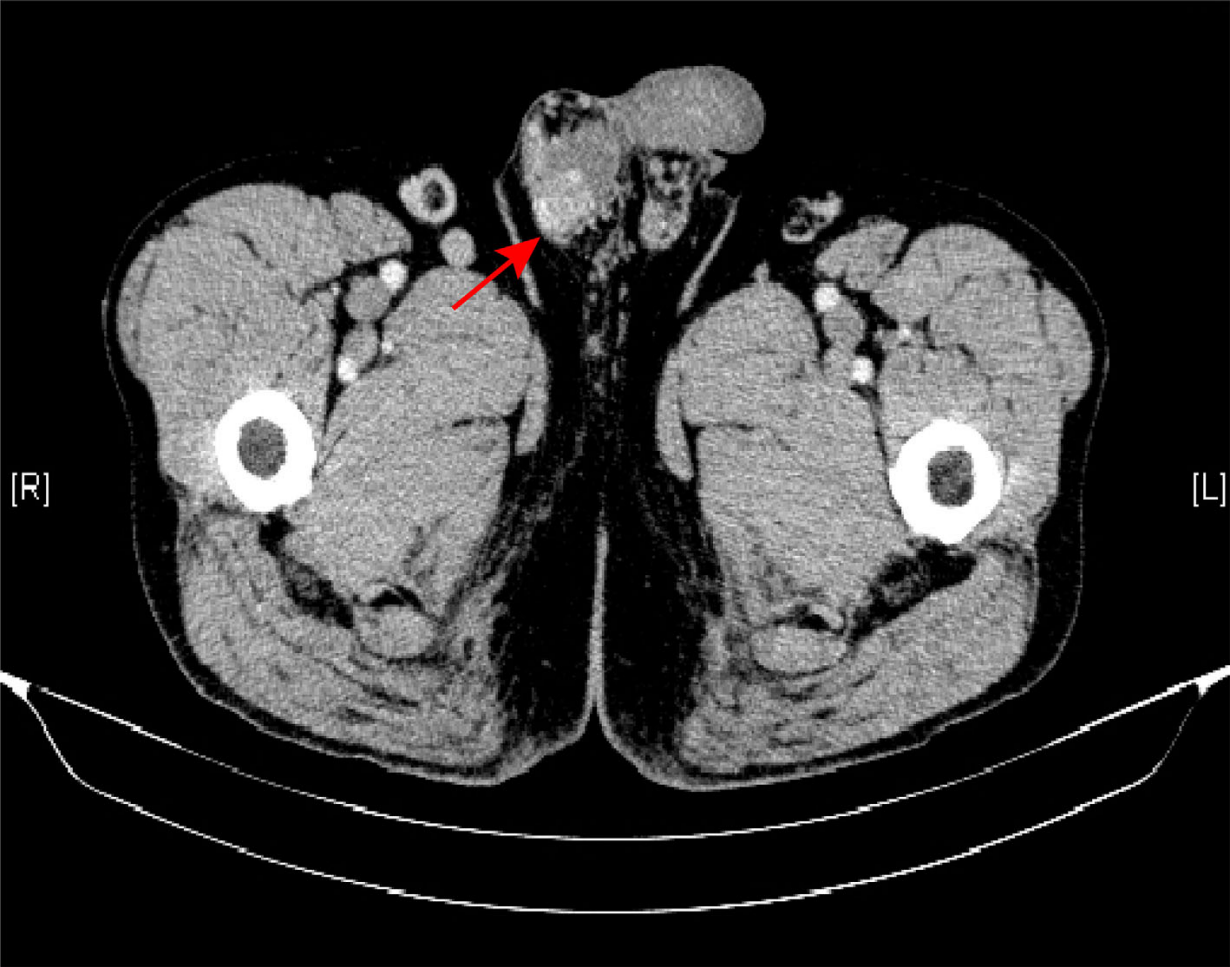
Enhanced computed tomography of the scrotum showed a nodular hyperdense shadow in the right epididymis (arrow).
